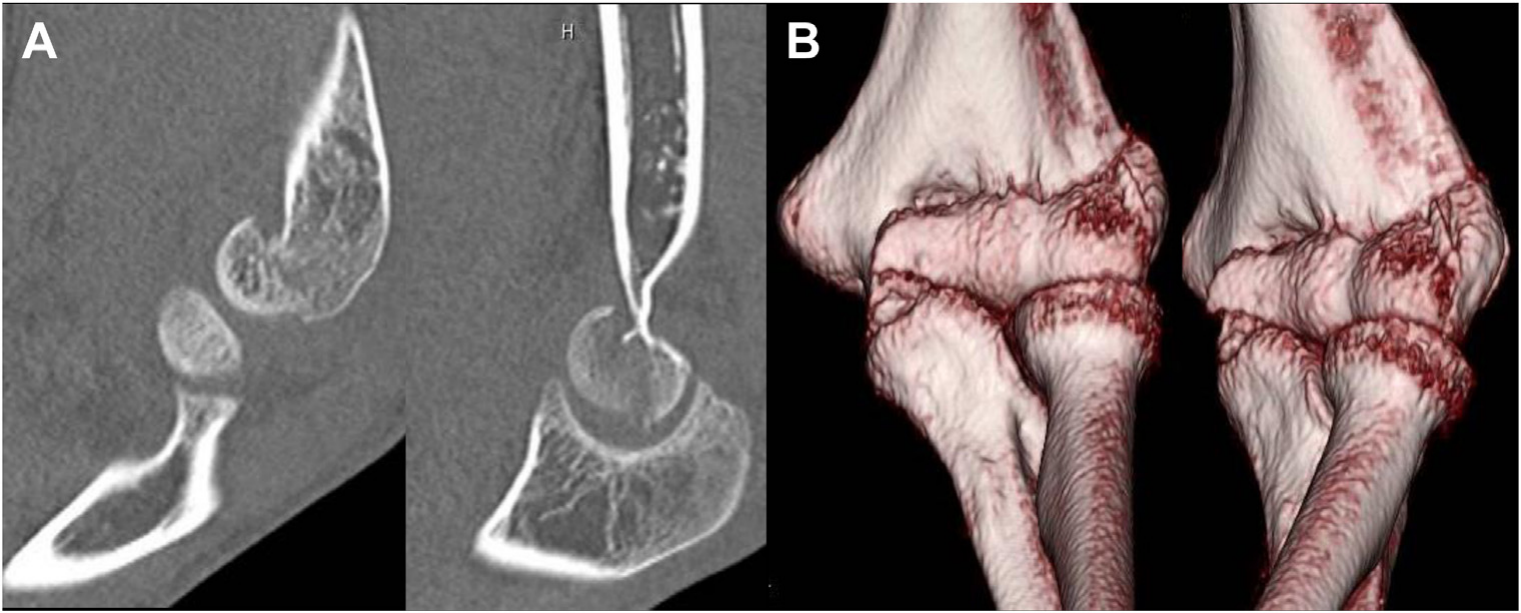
Computed tomography of the elbow joint at the first visit to our hospital. (A) Sagittal image and (B) 3-dimensional image. The distance of dislocation was 4 mm to the proximal side. The posterior humeral cortex was intact.
